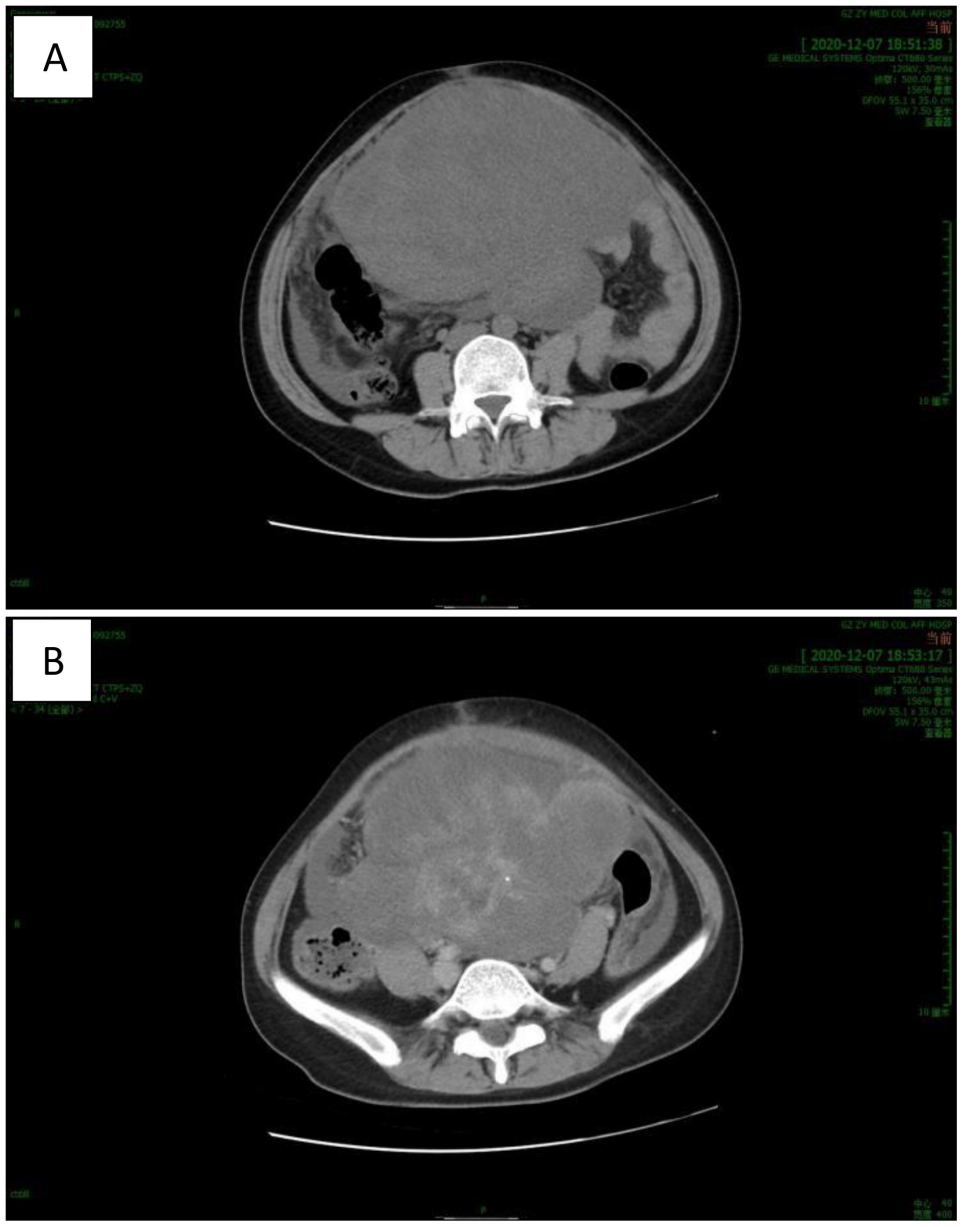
CT revealed a huge mass in the middle and lower abdomen with uneven density and septation. [(A) plain scan, (B) enhanced scan].
